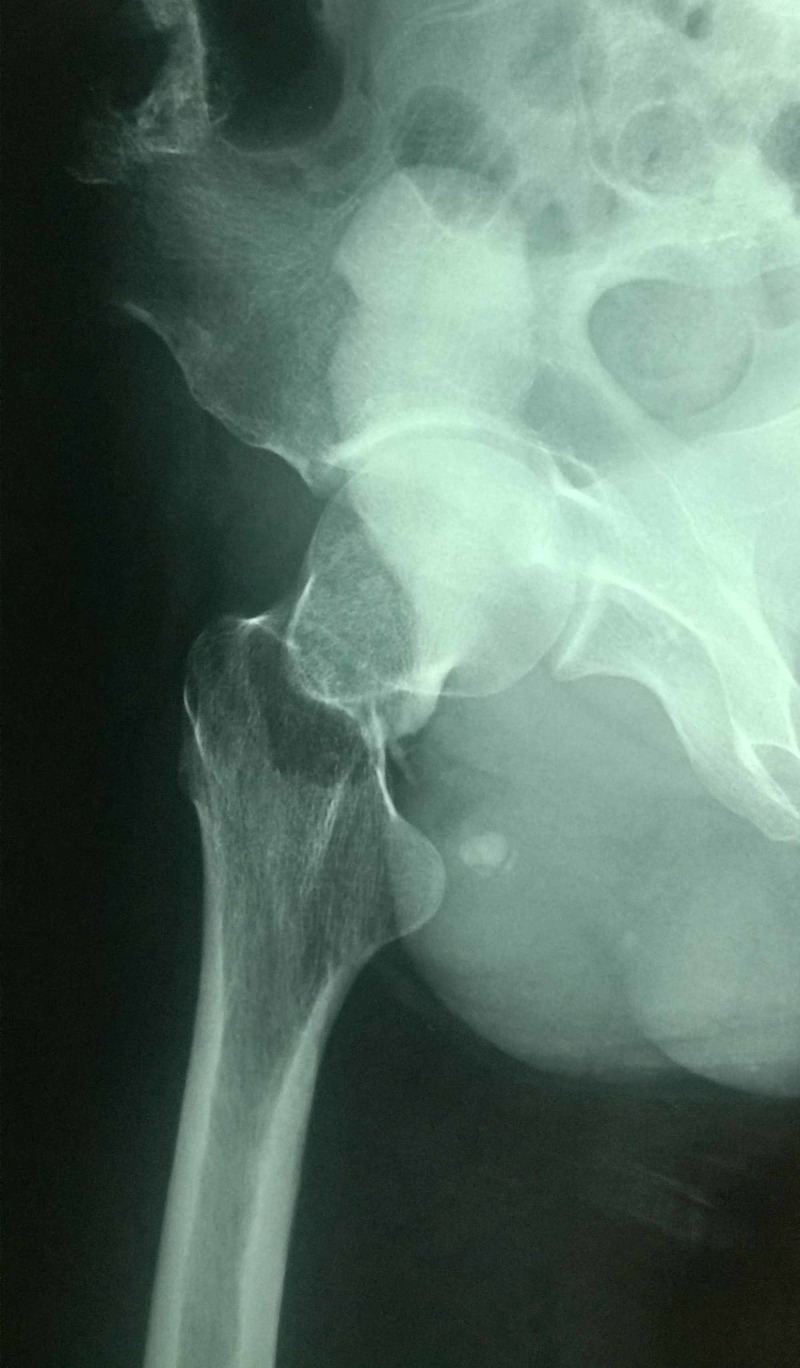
Lateral radiograph of patient A with the femoral neck fracture evident on it.
